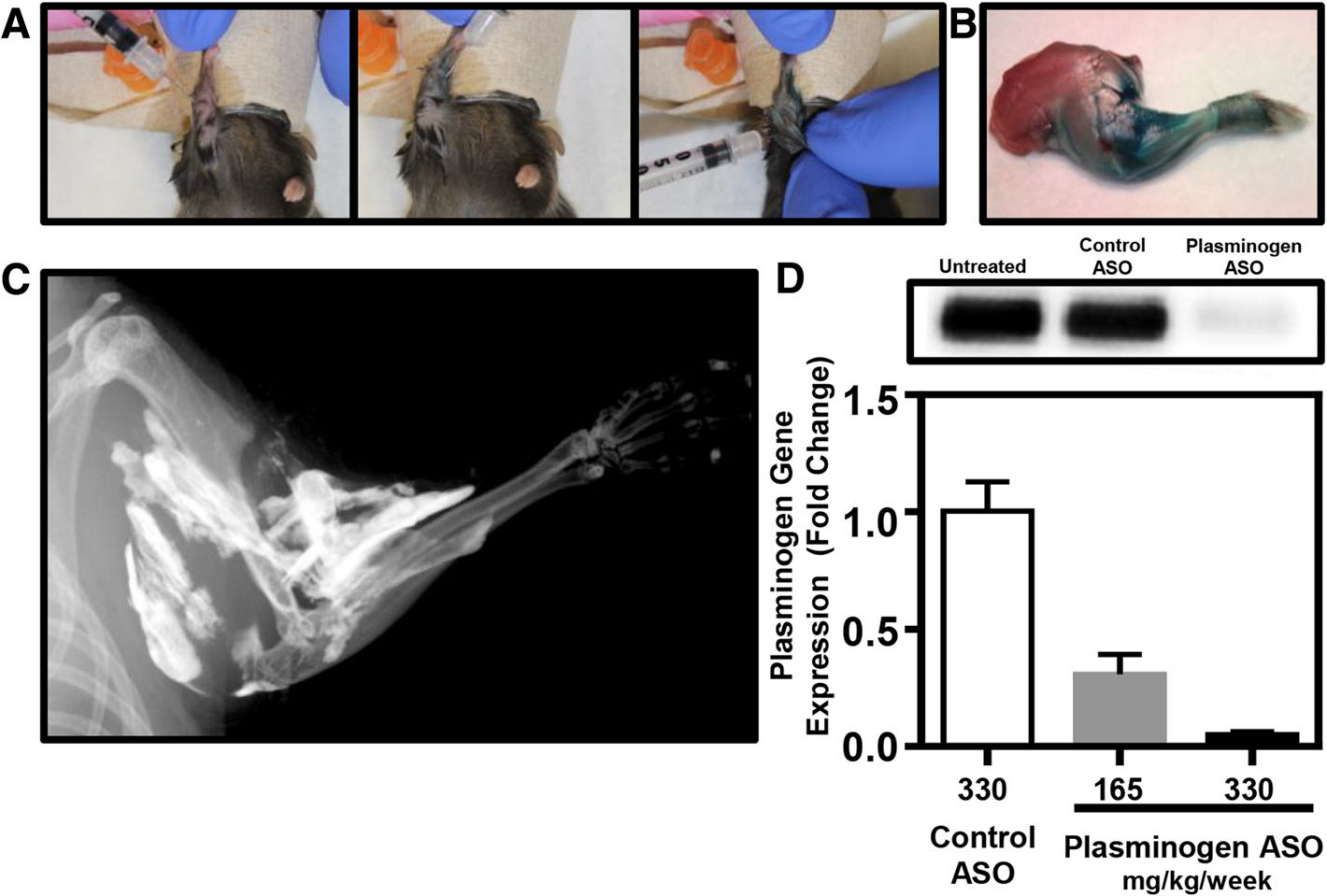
Preclinical Model of Upper Extremity Injury. a To reliably induce soft tissue injury around the elbow, three cardiotoxin injections were applied to the triceps, brachialis/brachioradialis, and the pronosupinators/hand extrinsics. b Injection of either blue dye or c) barium sulfate solution with subsequent radiographic analysis demonstrated well dispersed injection areas, fully surrounding the elbow joint and adjacent soft tissue. d Investigator induce plasminogen deficiency is attained by the time of upper extremity injury on both a protein (inset box) and RNA level following weekly administration of plasminogen ASO (330 mg/kg/wk) beginning two weeks before injury. Graphical representation of mean +/−SD
